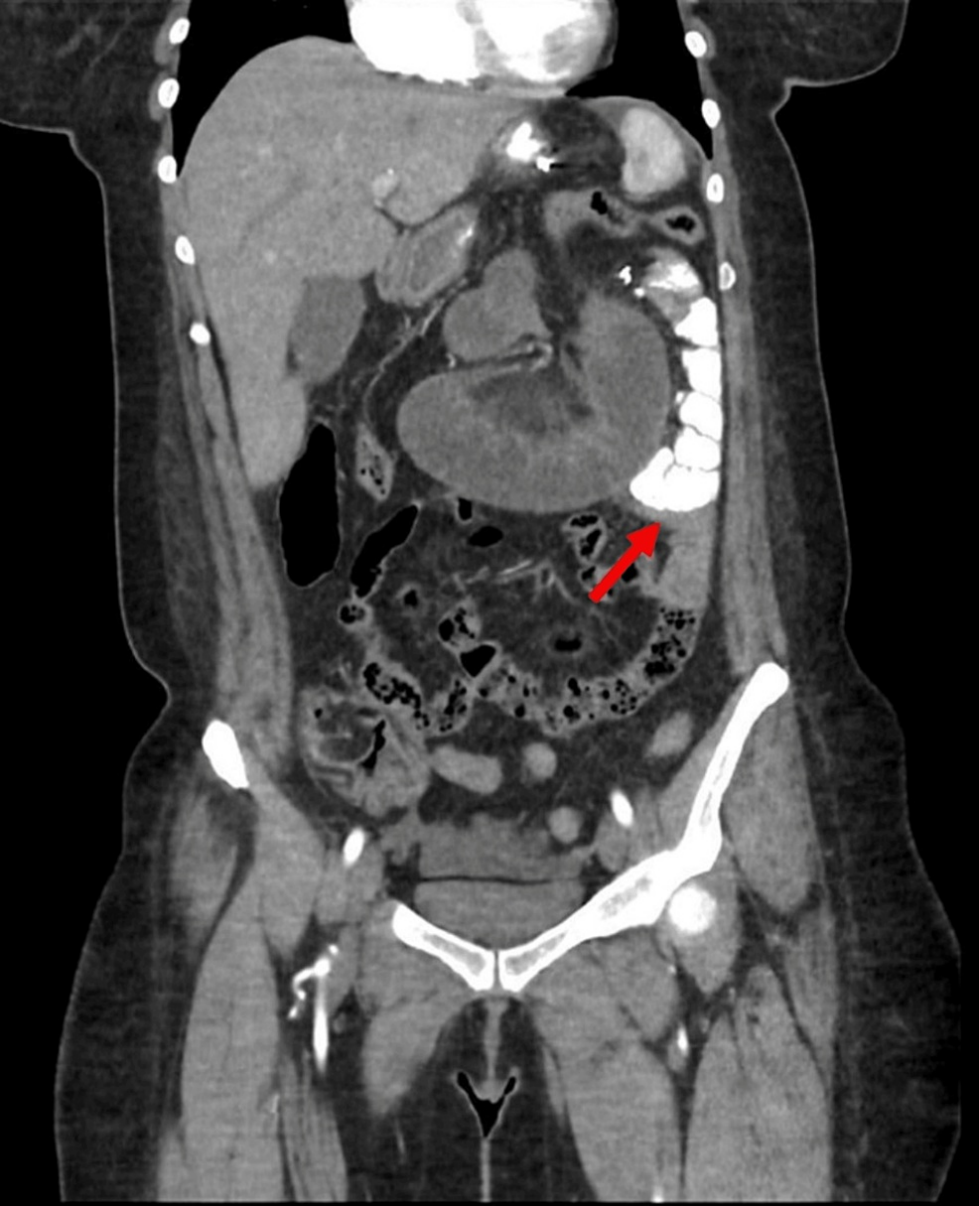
CT abdomen with contrast (coronal view) Showing signs of small bowel obstruction due to improper filling of the contrast, marked dilatation of parts of the small bowel surrounded by effusion
